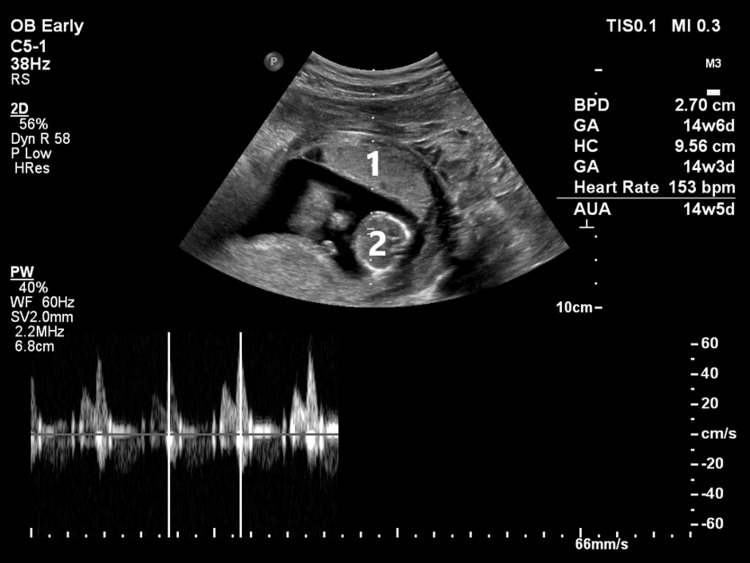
Bedside ultrasound image showing the anteriorly placed placenta with a small underlying hypoechoic area of retroplacental separation (1) and the cardiac area of an intrauterine viable fetus (2).
